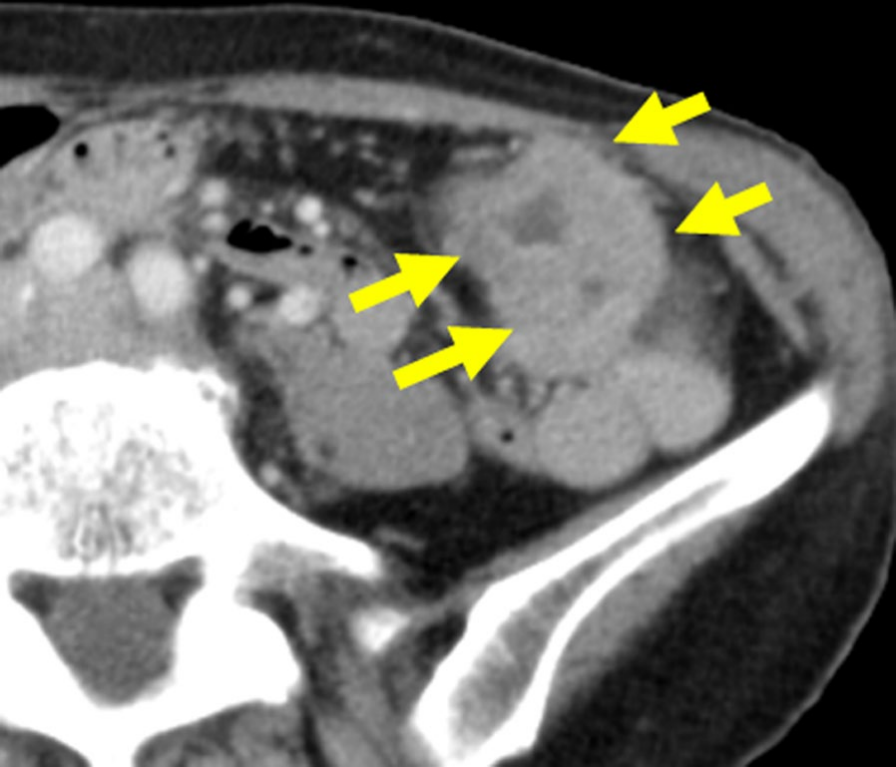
Contrast-enhanced abdominal computed tomographic scan showing sub-circular wall thickening of the transverse colon near the splenic flexure (yellow arrows)
